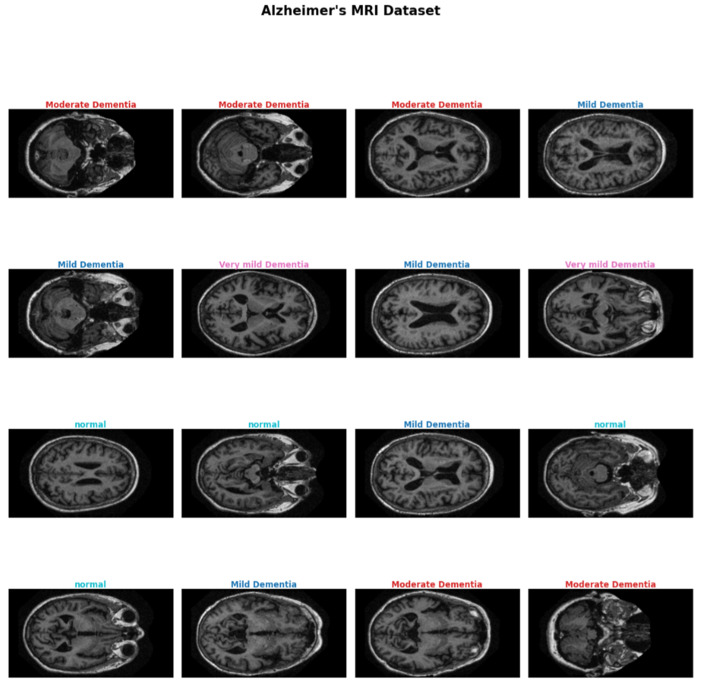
Images of brain MRI slices demonstrating the four stages of Alzheimer’s, consisting of normal, very mild dementia, mild dementia, and moderate dementia, showing atrophy of the progressive hippocampus and enlargement of the ventricles as the disease progresses.
